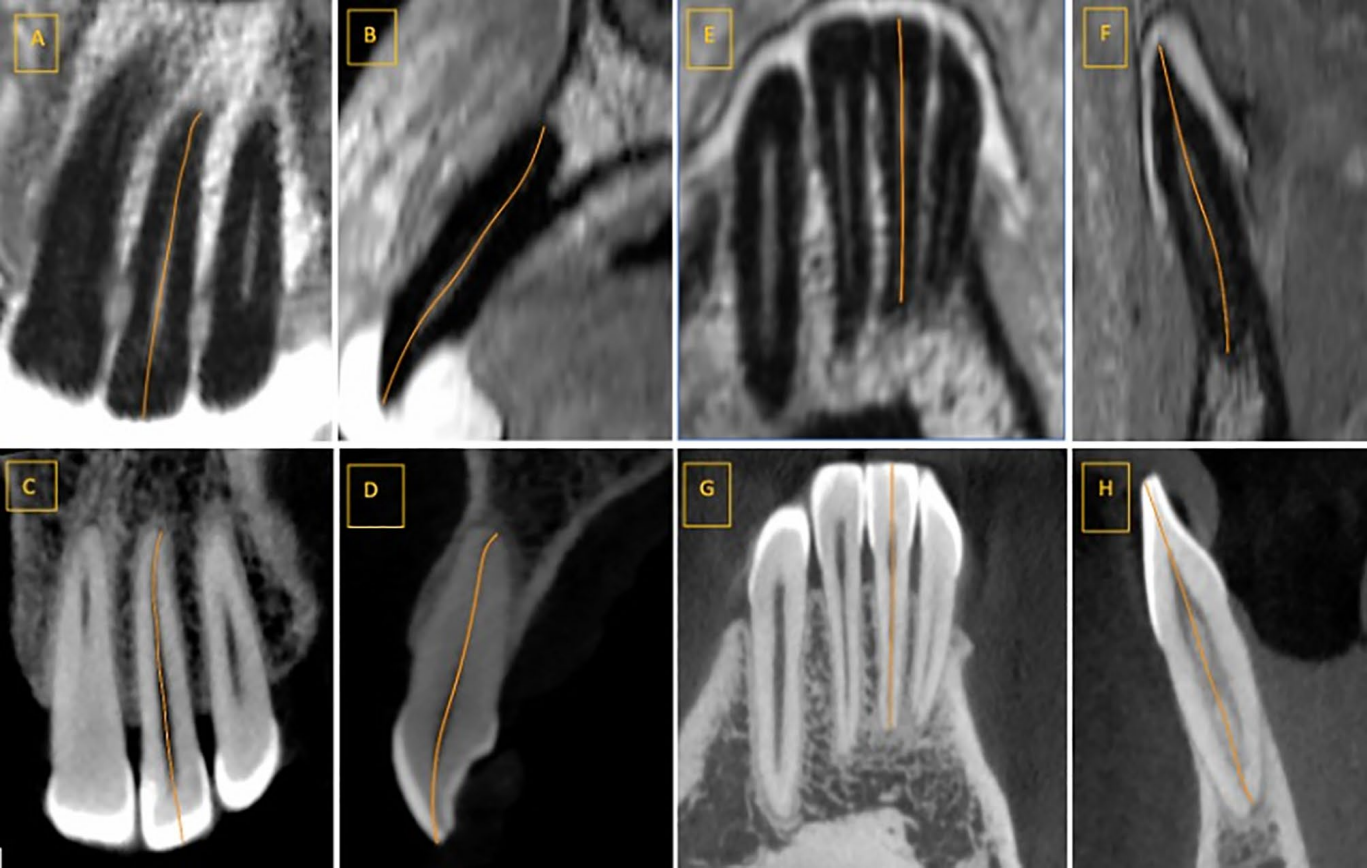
Canal length measurements by dMRI (upper row) and CBCT (lower row) in the mesio-distal (M-D) and vestibulo-oral (V-O) directions in the second left upper incisor (A–D) and the first right lower incisor (E–H). dMRI slices of a second incisor in the left upper jaw in the M-D direction (A) and the V-O direction (B). (C,D) Are the corresponding CBCT slices. dMRI slices of a first incisor in the right lower jaw in the M-D direction (E) and the V-O direction (F). (G,H) Are the corresponding CBCT slices.
